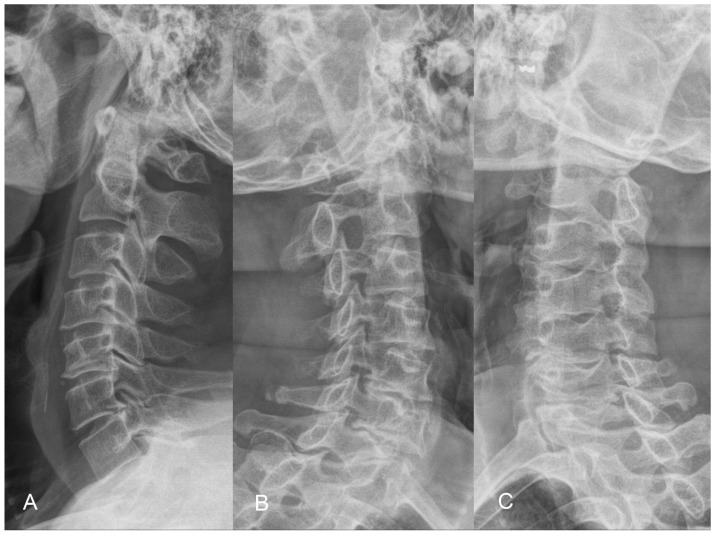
Preoperative lateral (A) and oblique radiographs (B,C) demonstrating well-maintained sagittal lordosis alignment and C3 to C7 foraminal stenosis, accompanied by the formation of osteophytes.
